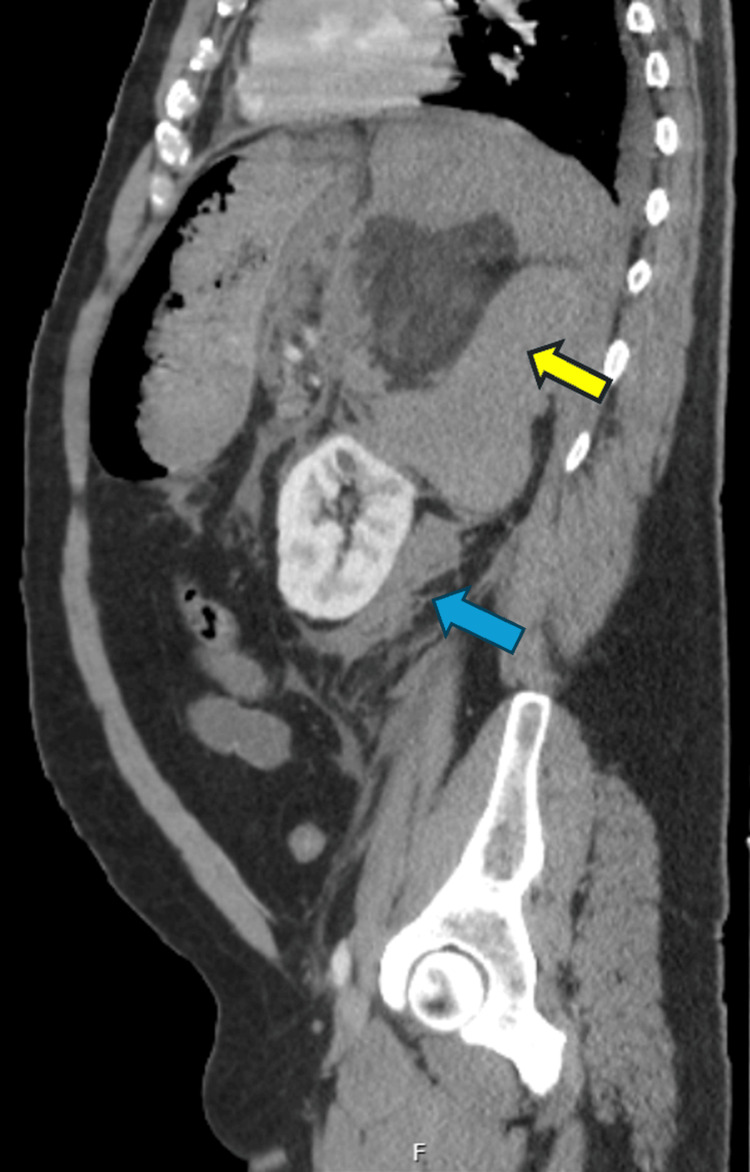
Renal angiomyolipoma Sagittal view of contrast-enhanced computed tomography of the abdomen and pelvis in the soft tissue window showing a large mass in the left kidney/suprarenal region containing soft tissue component, fat, and hemorrhage (yellow arrow). There is also evidence of moderate hemorrhage extending to the retroperitoneum, causing mass effect on the left kidney (blue arrow), spleen, and pancreas, concerning for a large angiomyolipoma with active hemorrhage to the right anterior pararenal space and pelvis.
